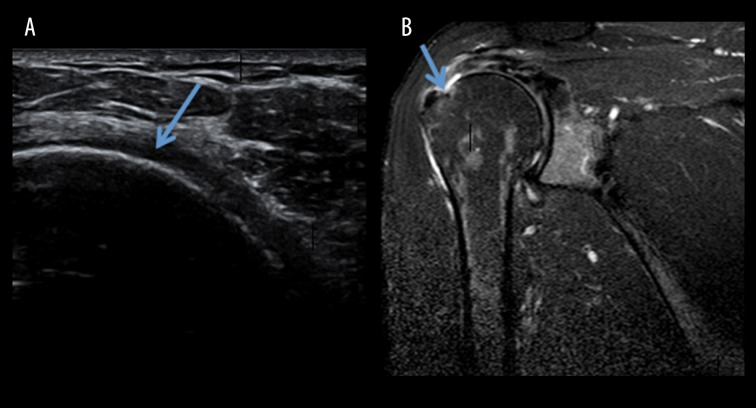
(A) USG image depicting a partial-thickness tear of the supraspinatus tendon (B) MRI, T2W coronal image depicting a partial-tear of the supraspinatus tendon (arrow marked).
